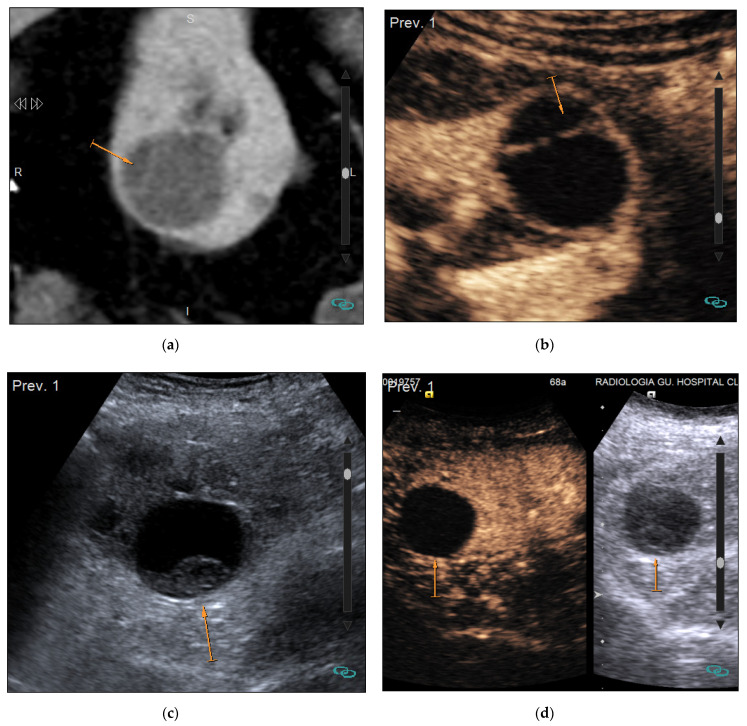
Evaluation of complex cysts with Contrast Enhanced Ultrasound (CEUS). (a) CT image shows a cyst with a thin septum (arrow) corresponding to a Bosniak II cyst in a 36-year-old woman; (b) CEUS image of the same cyst also shows the thin septum. Septa are usually better visualized by CEUS due to the possibility of real-time evaluation and its higher sensitivity for detecting microvascularization; (c) US image shows a complex cyst with suspicion of soft-tissue mass (arrow) in a 42-year-old woman; (d) CEUS image shows absence of enhancement of the intracystic lesion (arrow), corresponding to a Bosniak II cyst that does not require further examinations.
